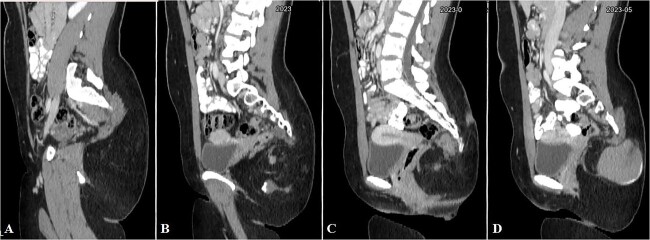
Selected sagittal CT cuts of the pelvis with oral and IV contrast (Venous phase) from left to right sides of the body (A–D), showing the mentioned gluteal mass. The cystic component of the mass is noted at the right gluteal region and the pre-coccygeal portion is well shown in C.
